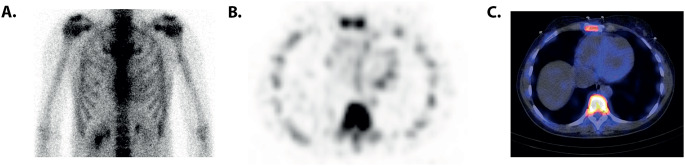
Bone scintigraphy showing Perugini grade 1 cardiac radiotracer uptake in an ATTRv amyloidosis patient with the p.(Ser43Asn) variant. A: anterior planar scintigraphy B: axial SPECT, and C axial SPECT/CT fusion. ATTRv = hereditary transthyretin; SPECT = single-photon emission tomography; CT = computed tomography
